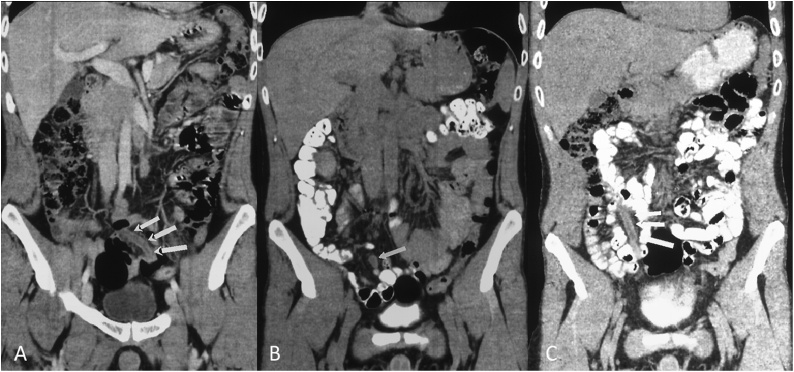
Coronal CT reconstructions after intravenous injection of contrast material (A) and hyperdense gastrointestinal contrast material administration (B and C) showing an hypodense tubular structure, 7 cm in length and 3 cm in width, strictly connected to a small bowel loop, with hyperdense thickened walls and perilesional fat strands into the adjacent mesentery (arrows).
